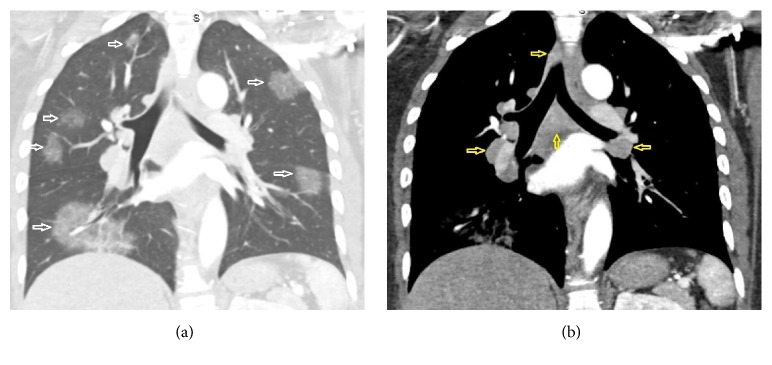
Coronal reformatted image from contrast-enhanced CT scan, with lung window (a), redemonstrating the findings in Figure 2 (white arrows), and with soft tissue window (b) demonstrating lymphadenopathy in the right paratracheal, bilateral hilar, and subcarinal regions (yellow arrows).
